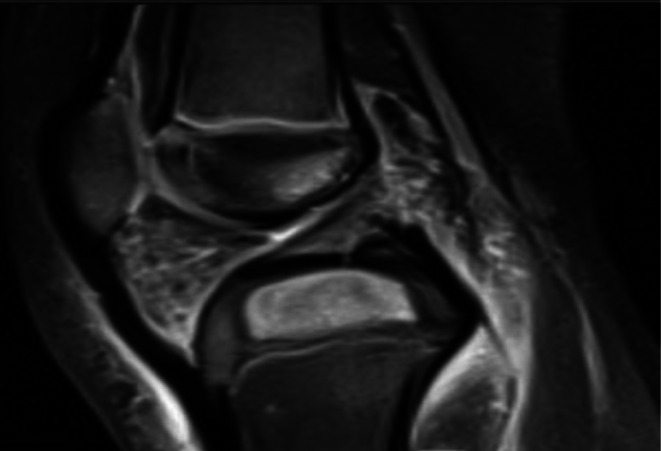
MRI showing an oval, heterogeneous enhancing lesion in the proximal tibial epiphysis.
